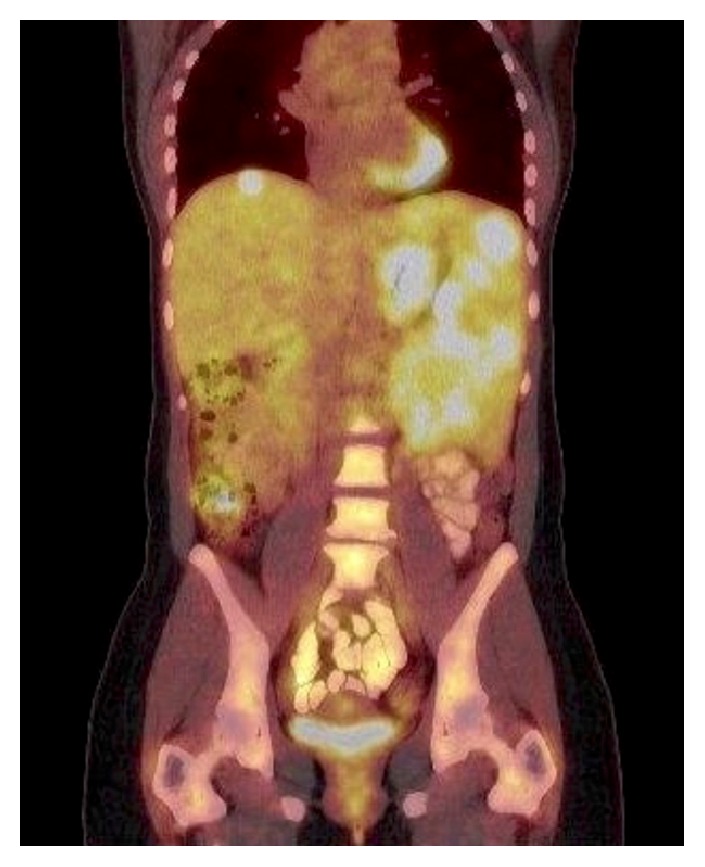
Preoperative PET-CT scan revealed multiple nodules in the spleen and a hepatic lesion with high metabolic activity.
